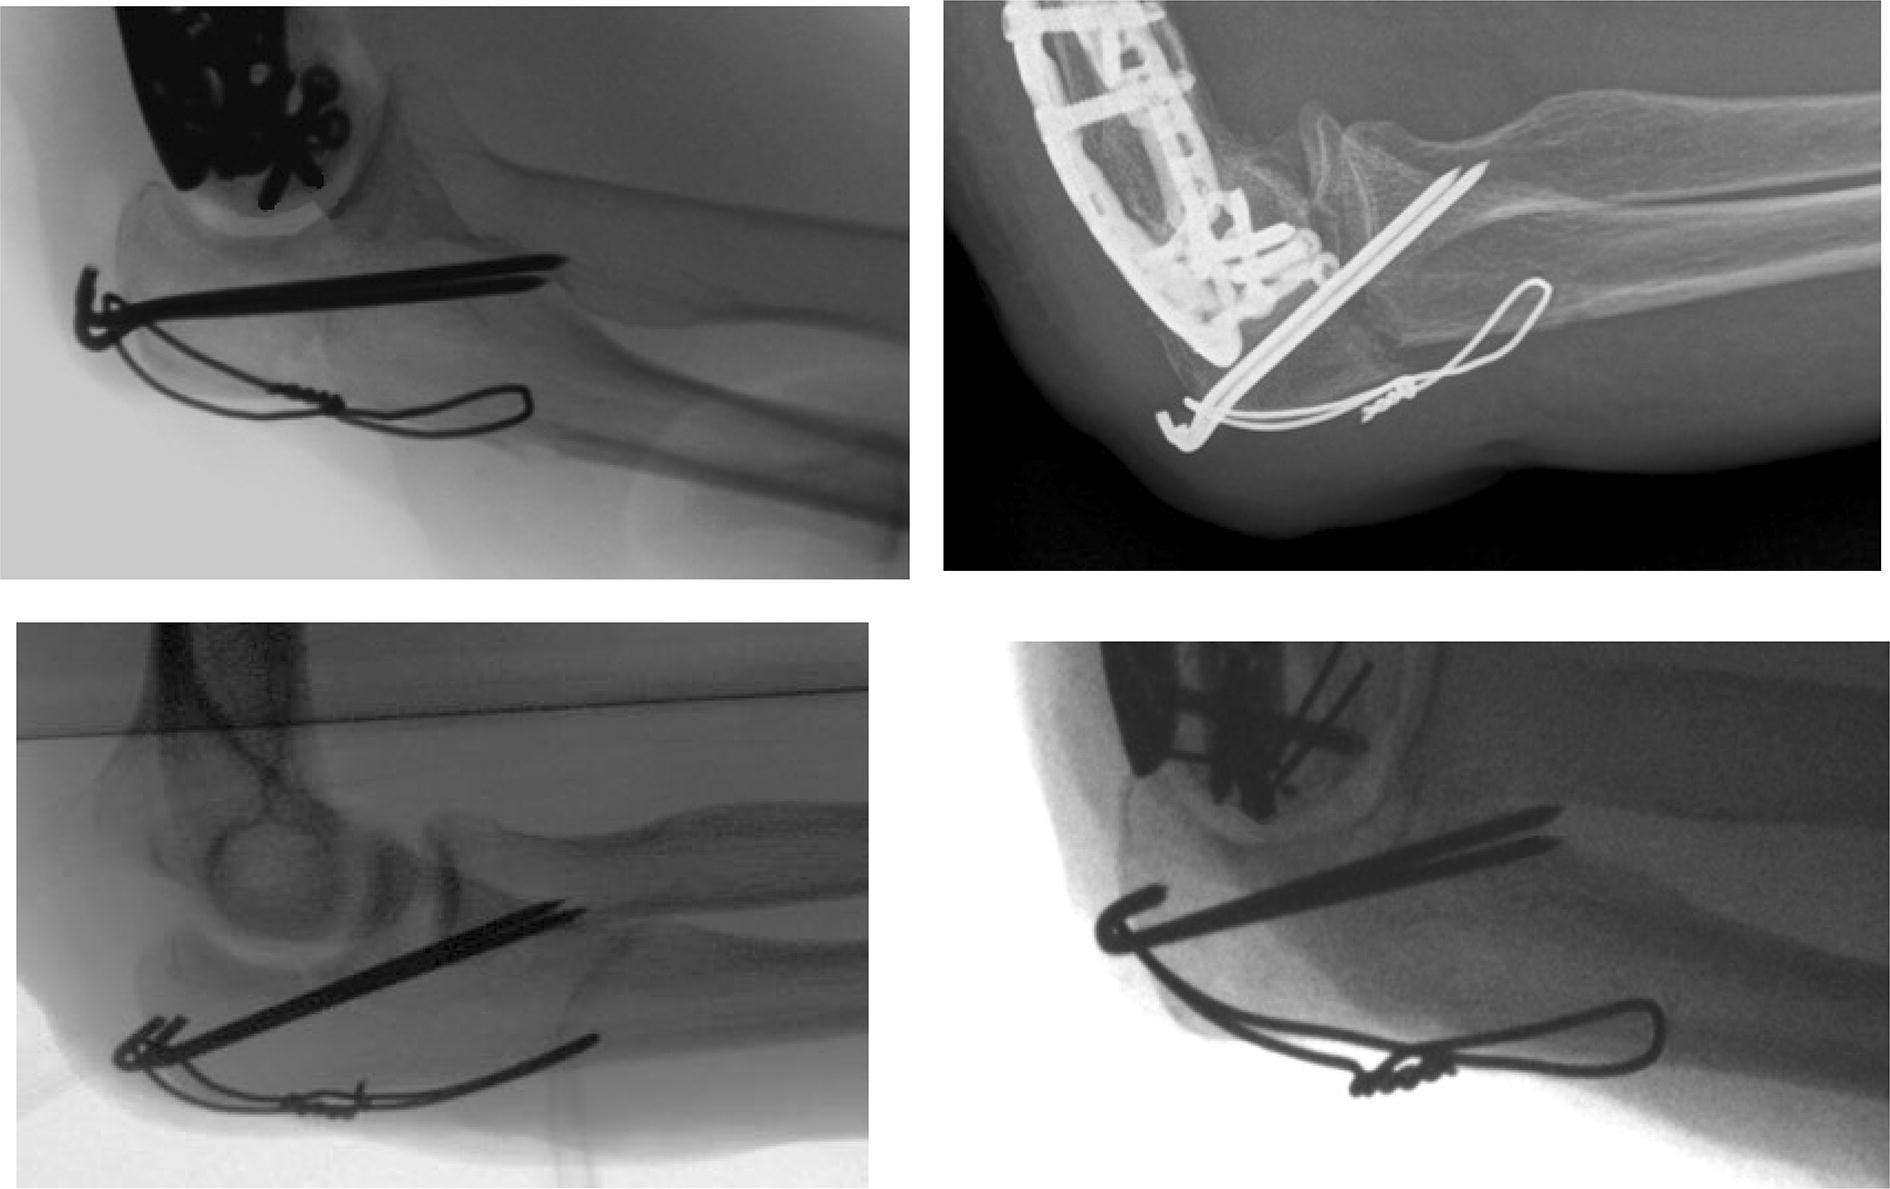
Clinical example of failure after bicortical fixation (top row) and regular X-ray after tricortical fixation (bottom row)
